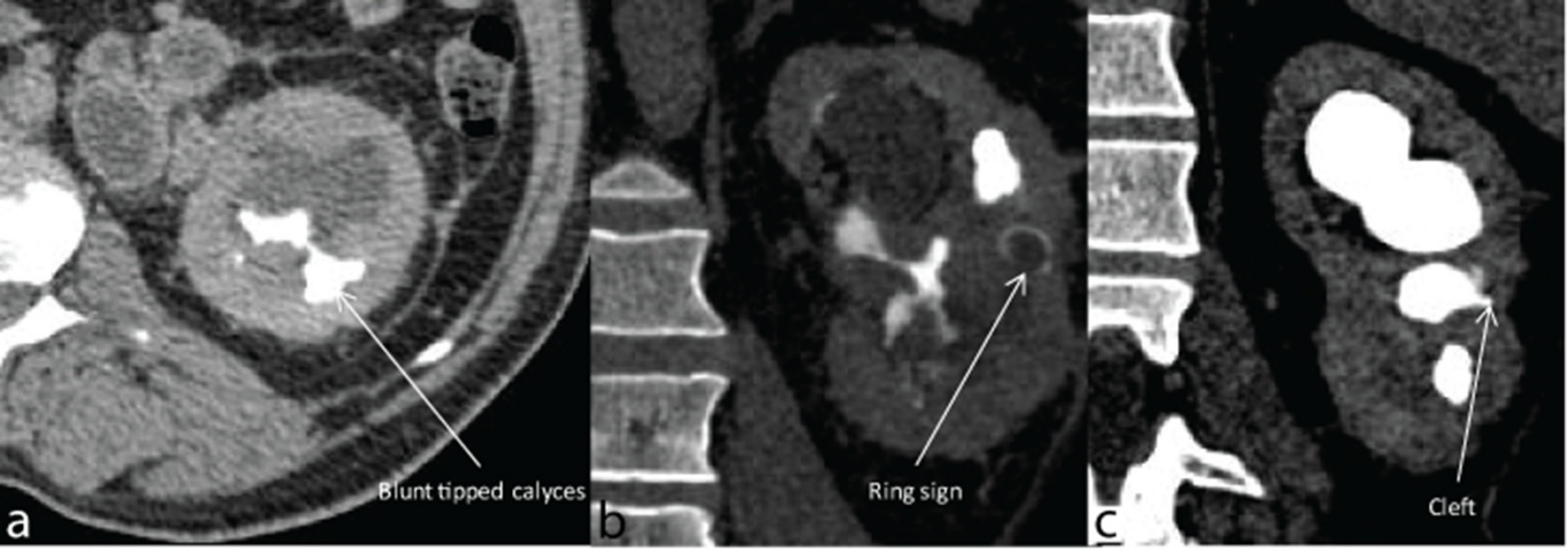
(a–c) Axial and coronal CT sections showing different signs of papillary necrosis: (a) blunt-tipped calyces in the right lower pole; (b) a ring sign, where a ring-like contrast opacification is noted around centrally non-opacified sloughed off papilla within the interpolar calyx. (c) Clefting in the middle interpolar calyx. In this coronal section pooling of contrast in dilated upper pole calyces is seen.
